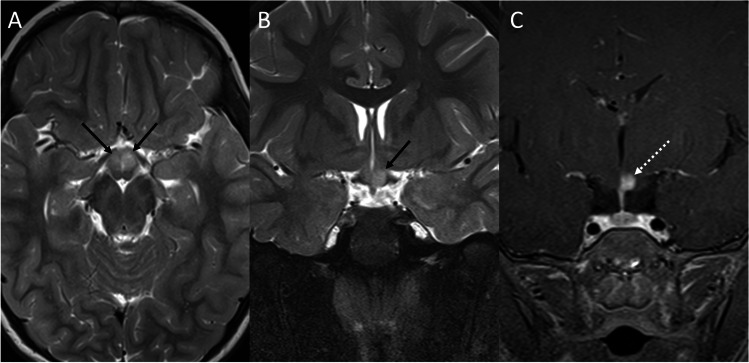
Hypothalamic glioma. Axial (A) and coronal (B) T2-, and coronal contrast-enhanced T1-weighted (C) sequences show a T2-hyperintense lesion (arrows) presenting a nucleus of enhancement (dotted arrow)
